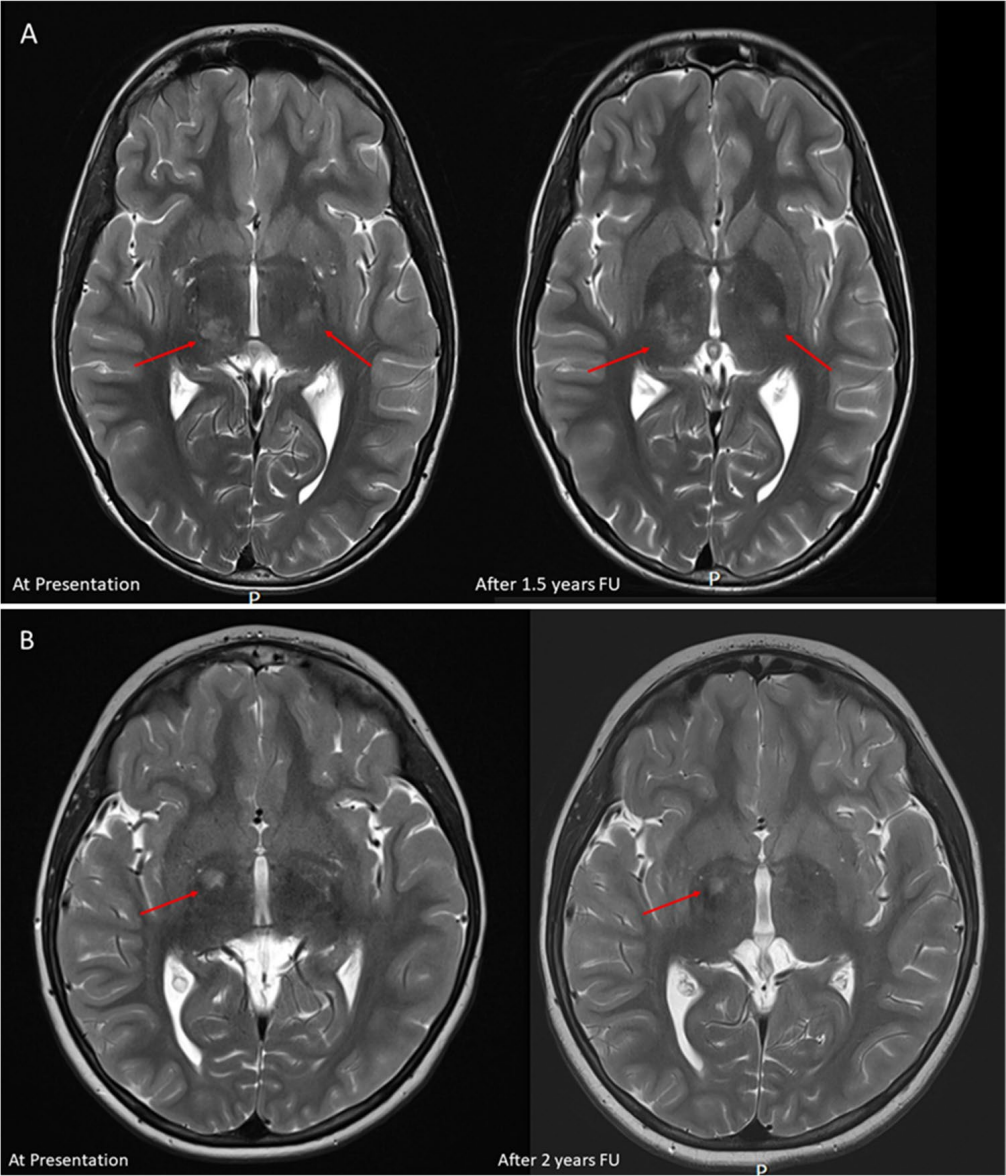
Examples of supratentorial incidental brain tumor findings managed conservatively A 12-year-old boy presenting with headaches. On MRI bi-thalamic incidental lesion. Conservative management with wait scan follow up was initiated, showing a stable lesion after 1.5 years. The boy remains neurologically intact B 7 year old girl presenting with scoliosis and torticollis. MRI showed an incidental finding in the right globus pallidus. Conservative management with wait scan follow up was initiated, showing a stable lesion after 2 years. The girl remains neurologically intact
